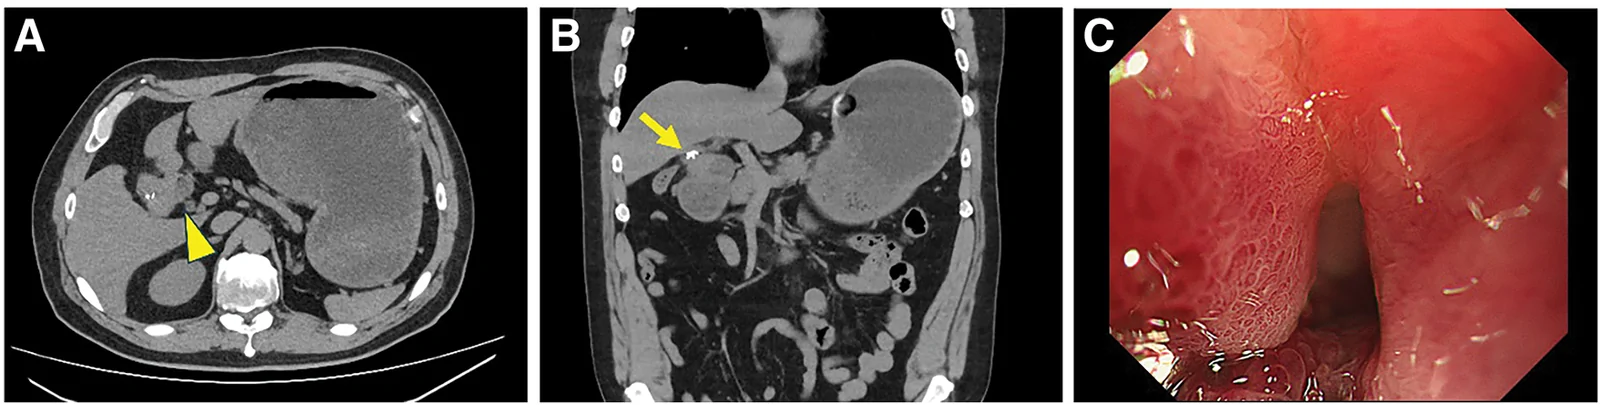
Contrast-enhanced abdominal CT and upper endoscopy images at the first admission. (A) The yellow arrowhead indicates the stenosis in the duodenal bulb. (B) The yellow arrow points to the clip placed on the CD during the prior cholecystectomy. (C) Endoscopic findings showing circumferential narrowing of the duodenum, although no obvious mucosal irregularity is observed. CD, cystic duct
